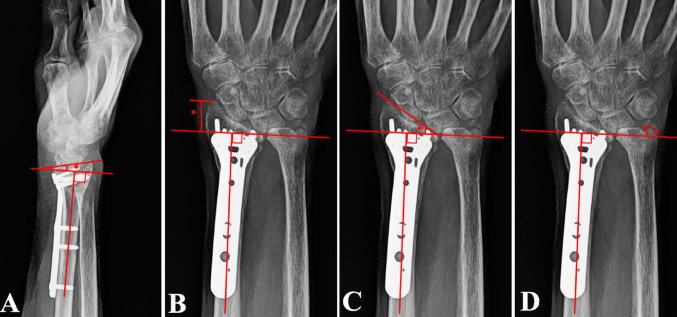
Standard radiographic measurements used to evaluate distal radius alignment: A volar tilt, B radial height, C radial inclination, and D ulnar variance
